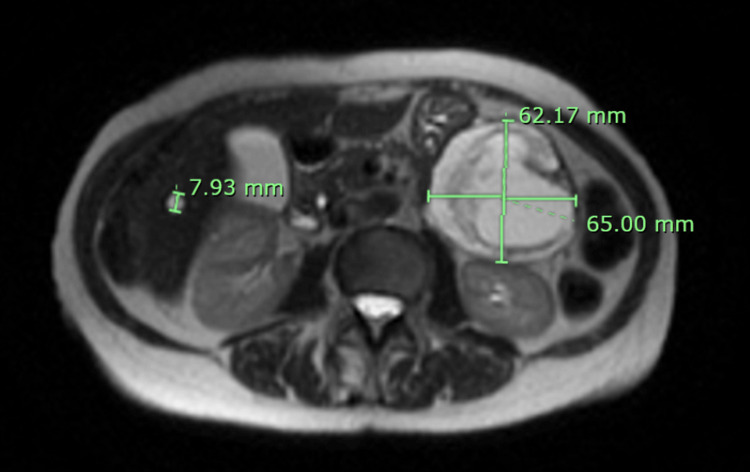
MRI appearance: axial SS-FSE sequence MRI of the abdomen without contrast (T2-weighted SS-FSE) demonstrating a complex mixed solid and cystic mass with peripheral enhancement and restricted diffusion, possibly arising from the pancreatic tail, which was suspicious for malignancy with no evidence of metastatic disease. SS-FSE, single-shot fast spin-echo; MRI, magnetic resonance imaging
